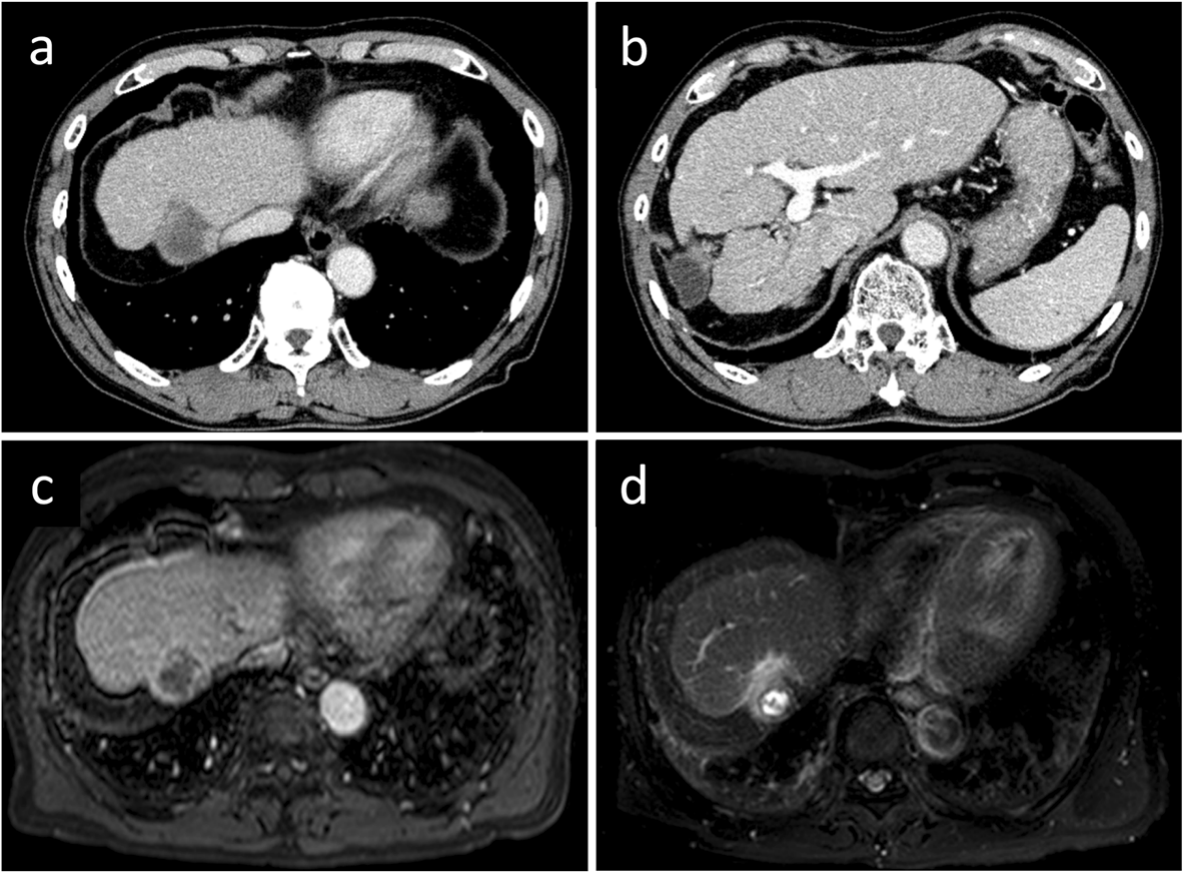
Enhanced CT and magnetic resonance imaging images before hepatectomy (54 months after the initial HAIC). Persistent tumor shrinkage with necrotic area, and no tumor thrombosis in the portal vein or the inferior vena cava, or intrahepatic metastases (a, b). Intratumoral necrotic area in the portal phase (c) and diffusion-weighted imaging (d)
